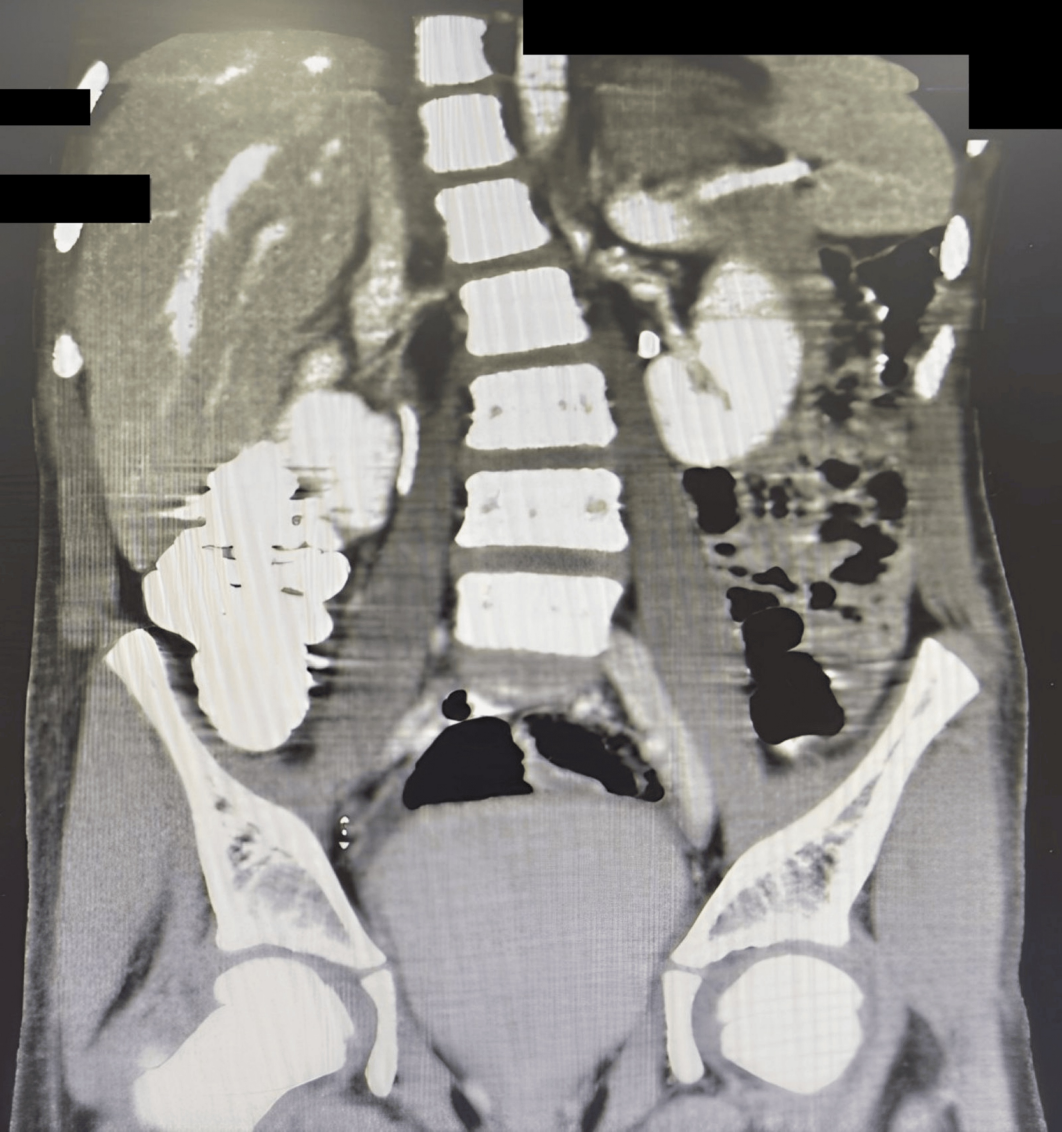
Coronal CT image showing an ill-defined fluid collection in the right iliac fossa, located between the terminal ileum and cecum, with mild mural thickening and faint peripheral enhancement
